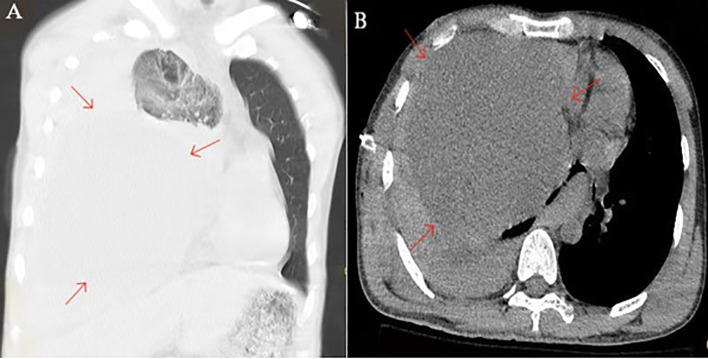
CT imaging.Coronal (A) and axial (B) images features of solitary fibroma (red arrows).
